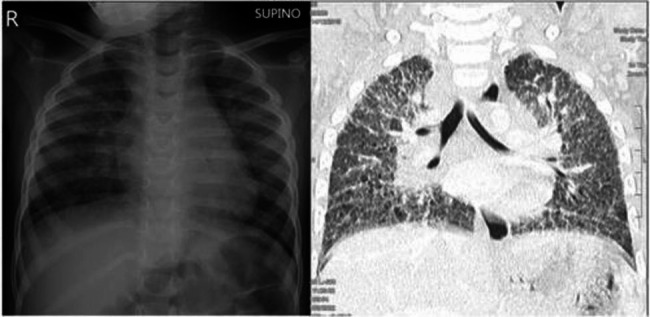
Chest X-ray with diffuse interstitial thickening (a); pulmonary CT with diffuse marked thickening of the pulmonary interstitium with ground-glass areas especially at the lower lobes. At the subpleural level, bilaterally, tiny cystic formations associated with bronchiolectasias (b)
